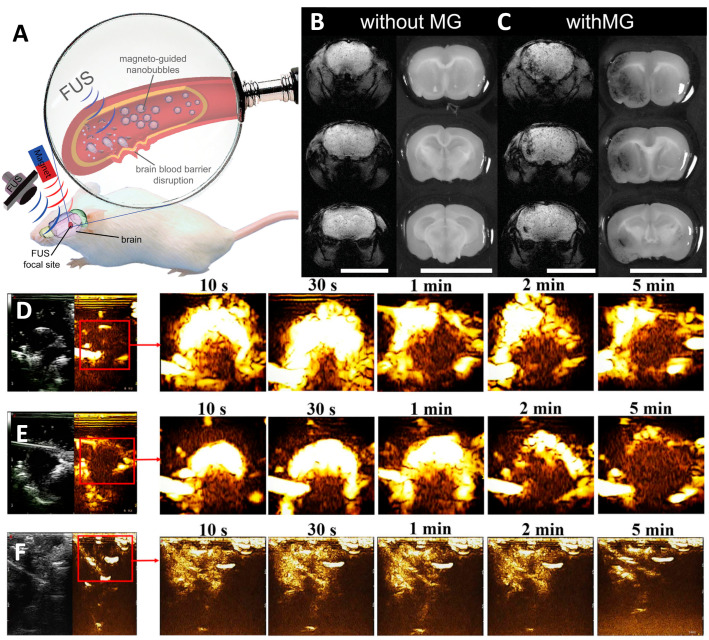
(A) Nanobubbles can be used to diagnose locally disrupted BBB and accumulate due to magnetic guidance (MG). (B) Representative images of T2 gradient brain slices and their corresponding dye-treated brain tissue slices, used to assess the efficiency of bubble-free and magnetically guided brain tissue BBB disruption. (C) Biosafety induced by focused ultrasound compared to different degrees of BBB destruction caused by nanobubbles. Contrast-enhanced ultrasound imaging of brain tumors at other timepoints (10 s to 5 min) before and after injection of (D) nanobubbles and (E) commercial SonoVue. (F) Brain cavity ultrasound images at the same timepoint before and after the injection of NBs. Red squares show image enhancement of specific tissue sites. Adapted with permission from Refs. [103,104]. Copyright 2020 Elsevier and 2014 John Wiley and Sons.
